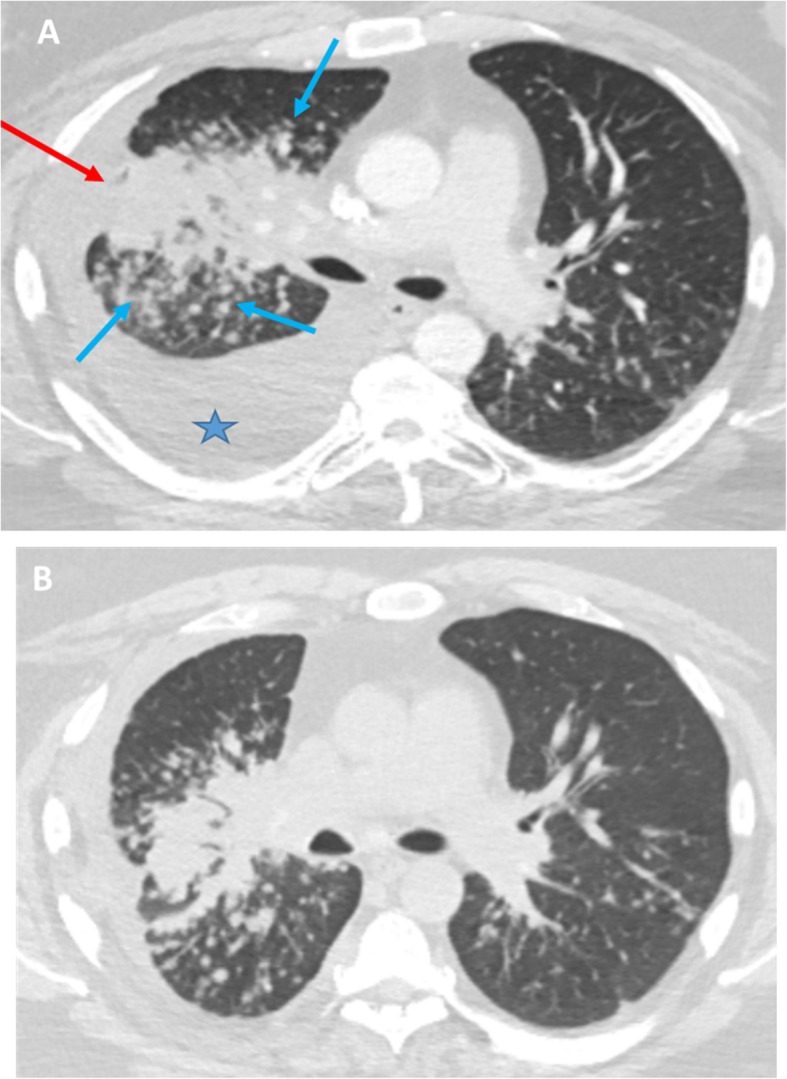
a Axial chest computed tomography image at presentation demonstrating a large area of right mid lung consolidation (red arrow) with adjacent extensive nodularity (blue arrows) along with a large right plural effusion (star). b Follow up Chest computed tomography after 8 months of prednisone administration results in significant improvement of right lung infiltrates as well as resolution of right sided pleural effusion
